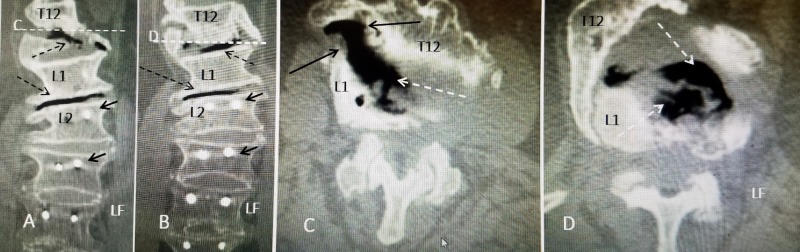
CT reconstruction comparing coronal and axial images showing extensive vacuum changes in the inter-vertebral disc space that are clearly extending into the avulsion fracture. A: Coronal CT reconstruction showing the level of axial slice 'C' (fine dashed white line with C). The vacuum change is seen within the avulsed fracture of T12 and T12-L1 and L1-L2 disc spaces (dashed black arrow). The pedicle screws at L2 and below are indicated (solid black arrow). Left is indicated (LF). B: Coronal axial CT showing axial slice 'D' (fine white line with D). The osteonecrosis and CT vacuum changes in T12-L1 and L1-L2 disc spaces are marked (dashed black arrow). The pedicle screws at L2 are indicated (solid black arrow). Left is indicated (LF). C: Axial CT made at the level of ventral osteophyte between T12 and L1 just below slice 'C'. There is vacuum signal change in the disc space (dashed white arrow) that extends both ventral and lateral into the fractured osteophyte between T12 (solid black arrow) and inferior edge of osteophyte at L1 (solid black arrow with white dots). D: Axial image showing vacuum change primarily within the disc space at T12 and L1 (dashed white arrow). Left is indicated (LF).
